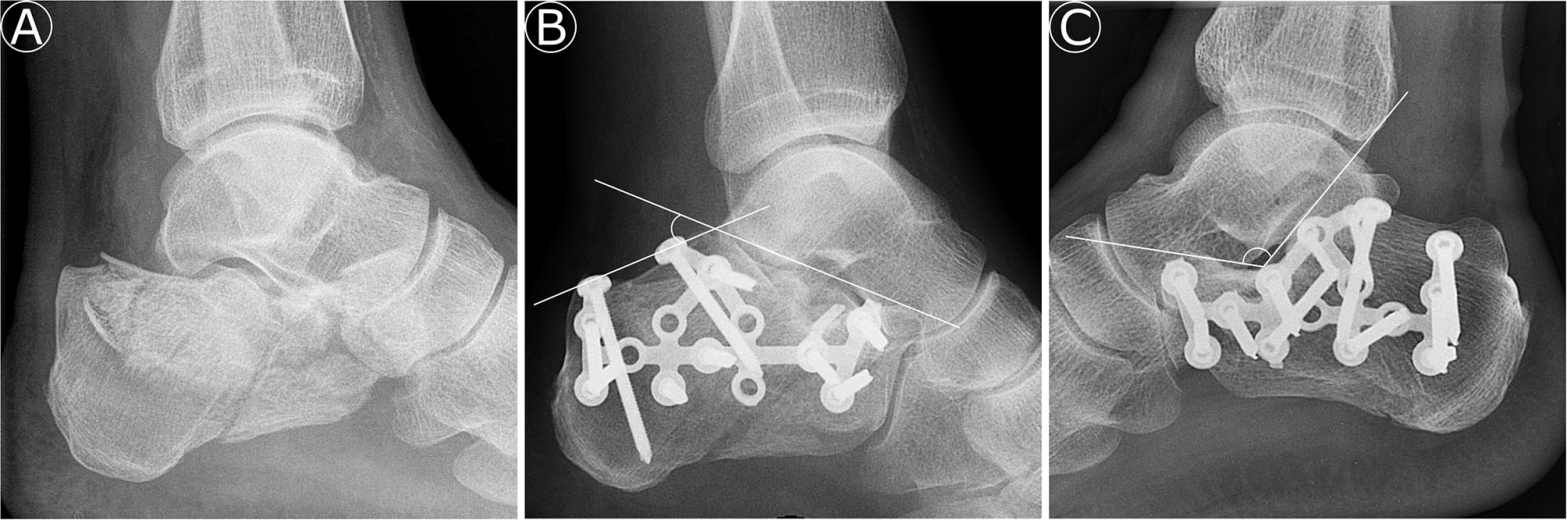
(A) Lateral preoperative radiograph of a Sanders-type 3AC calcaneal fracture. (B) Lateral postoperative radiograph of the calcaneal fracture from (A) with improved plotted Bohler's angle (39°). (C) Lateral postoperative radiograph of a calcaneal fracture with a marked Gissane angle of 123°.
